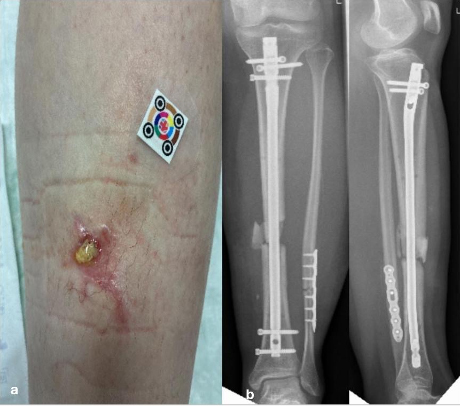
(a) A 16-year-old patient with a lower leg fracture classified as Gustillo–Anderson grade III. Initial management involved external fixation, followed by definitive fixation with intramedullary nailing. The patient is currently presenting with a fistula and a fracture-related infection (FRI), often misinterpreted as wound healing disturbance. (b) Anteroposterior and coronal radiographs demonstrating a lower leg fracture stabilized with intramedullary nailing. The images reveal a non-progressive fracture healing pattern with radiological features consistent with fracture-related infection.
